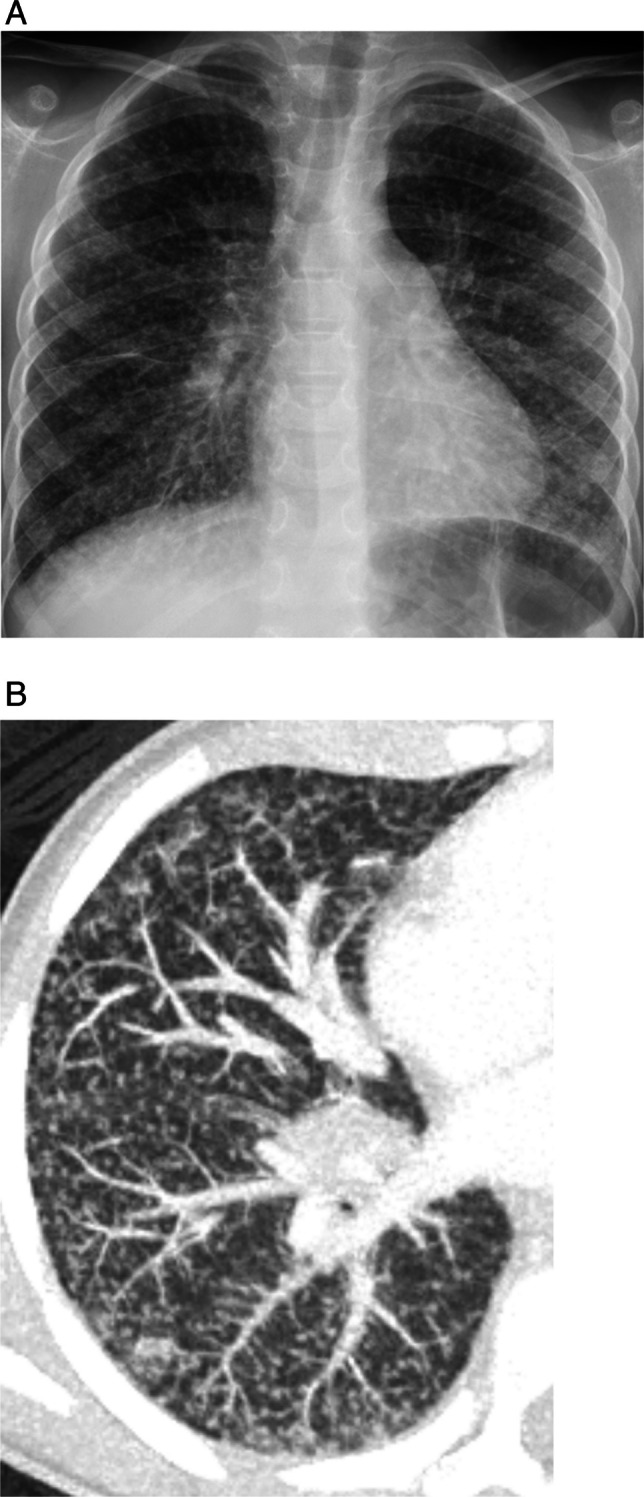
Acute disseminated histoplasmosis in a 6-year-old male presenting with worsening cough and fever. A Frontal chest radiograph demonstrates miliary nodules, (B) better visualized on CT with contrast enhanced axial MIP
